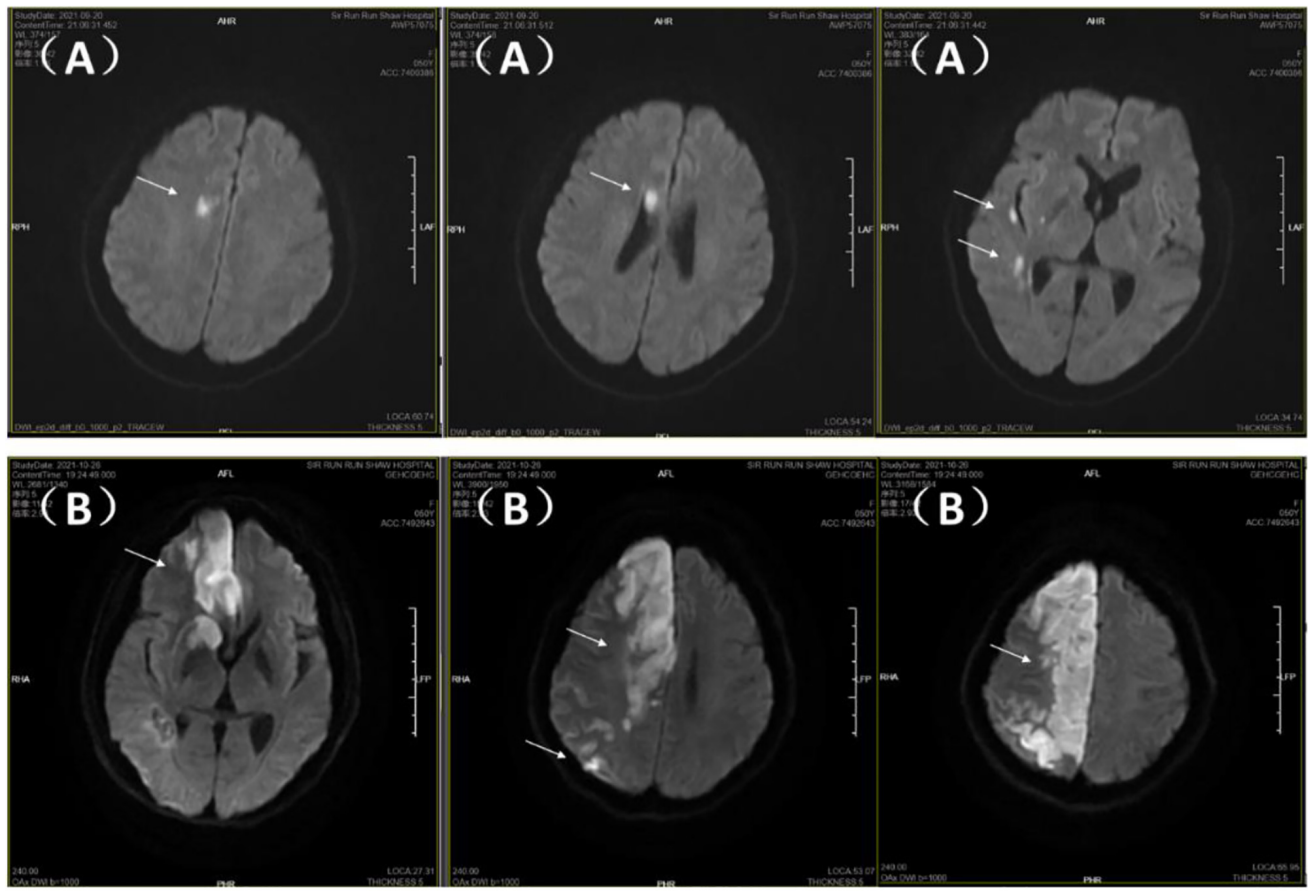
Brain MRI-DWI sequences. (A) Initial admission: multiple small infarcts in right frontotemporal region, basal ganglia, and periventricular area (arrows). (B) Readmission: progression to extensive right hemispheric paramedian infarction (arrows).
